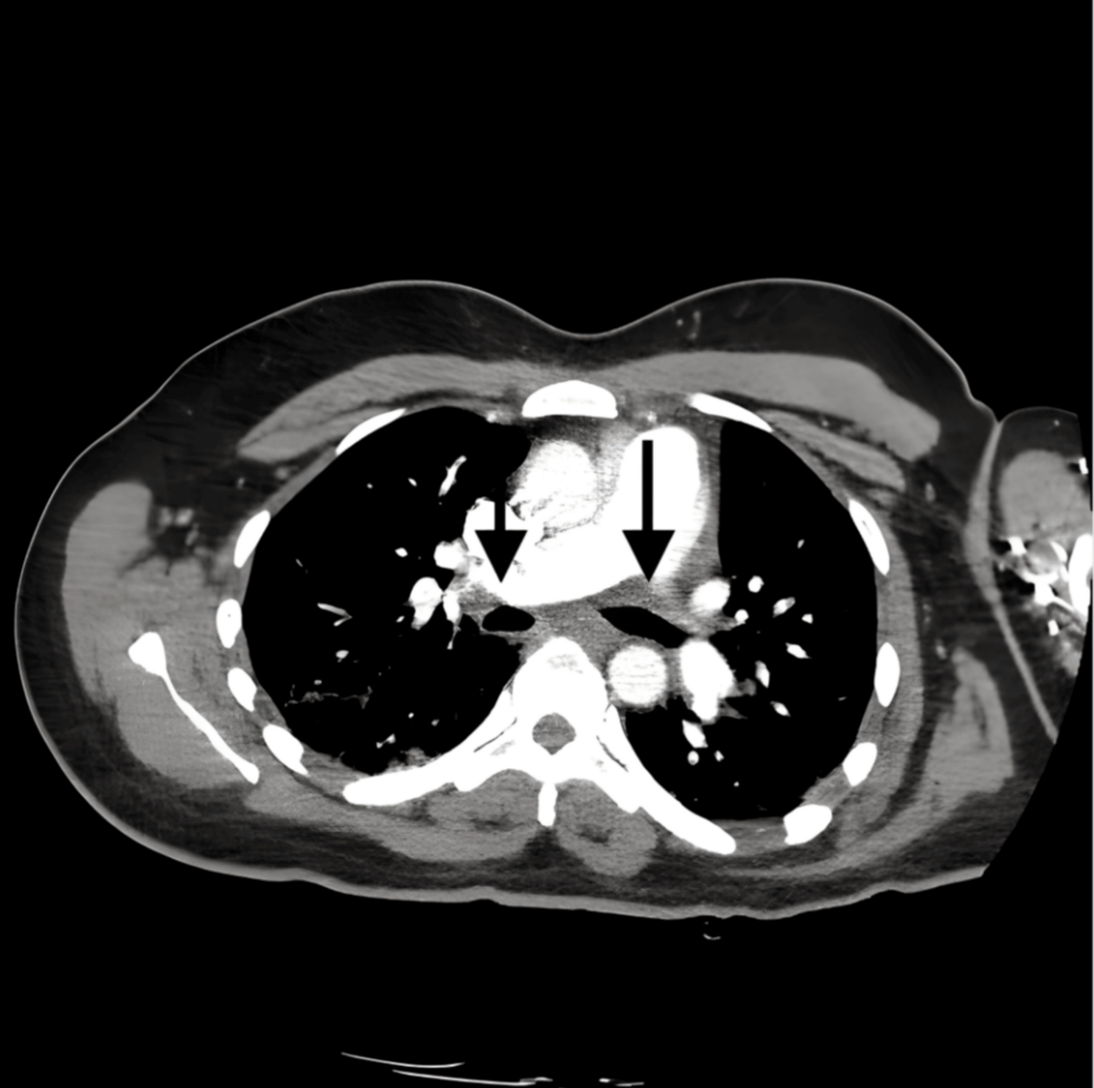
CT chest with intravenous contrast: axial view. This image demonstrates normal contrast opacification of the central pulmonary vasculature. Arrows: The pulmonary arteries are patent with no evidence of intraluminal filling defects, consistent with the absence of pulmonary embolism.
